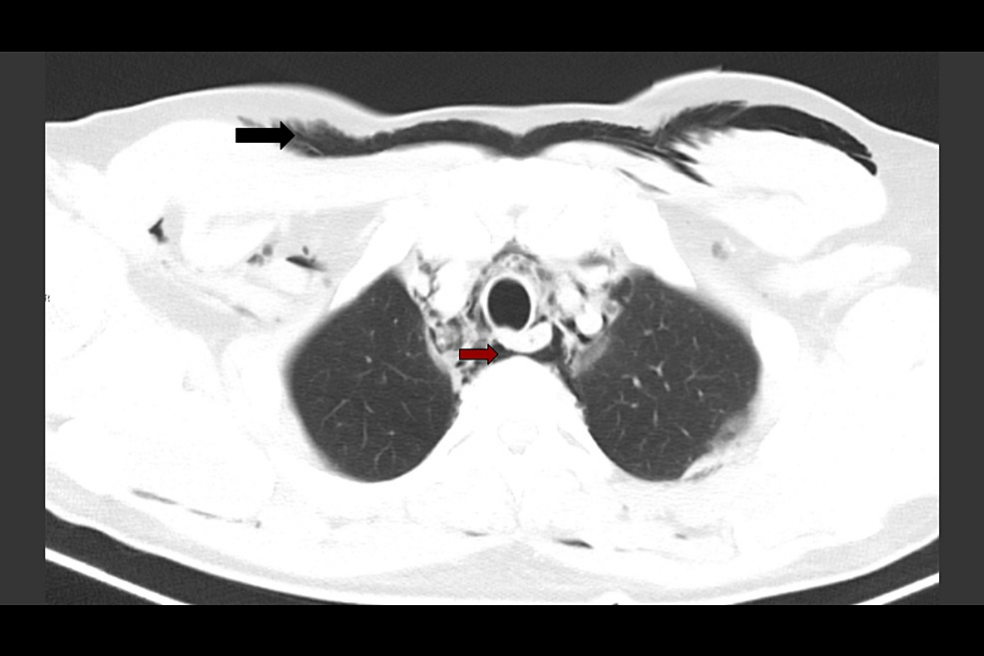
CT scan of chest day 3: axial slice showing air from mediastinum has dissected posteriorly (red arrow) to the esophagus.
